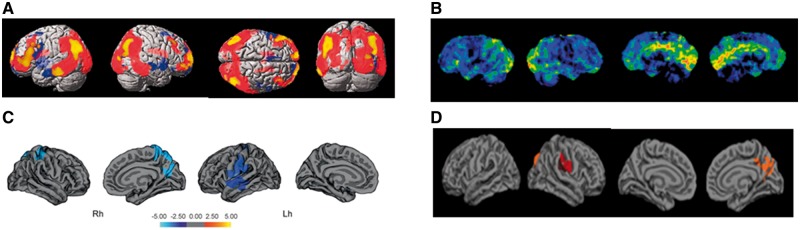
Cerebral hypometabolism and dementia in Parkinson’s disease and grey matter atrophy in Parkinson’s with cognitive involvement. (A) Regions of cerebral hypometabolism in patients with PDD overlap with regional atrophy. Adapted from González-Redondo *et al.* (2014). (B) Statistical maps of baseline ^18^F-FDG-PET data comparing patients with Parkinson’s disease that later develop dementia with controls. Hypometabolism is seen in posterior brain regions particularly in cuneus and precuneus. Image adapted from Bohnen *et al.* (2011). (C and D) Vertex-wise comparisons of cortical thickness between patients with PD-MCI and Parkinson’s disease without cognitive involvement. Atrophy patterns differ between studies, although atrophy in the precuneus is frequently reported. (C) Greatest atrophy seen in left precuneus. Modified with permission from Pereira *et al.* (2014). (D) Greatest atrophy seen in precuneus and bilaterally in superior parietal regions. Figure adapted from Segura *et al.* (2014). Lh = left hemisphere; Rh = right hemisphere.
